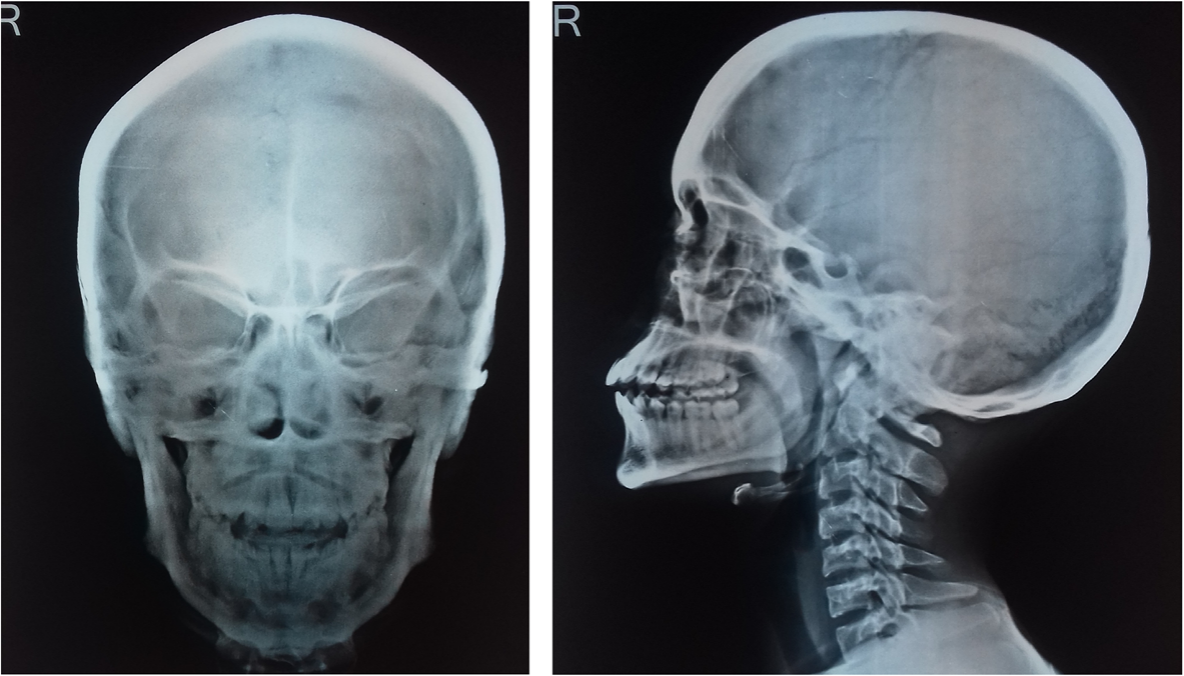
Radiography of skull showed mild cortical and subperiosteal thickening
